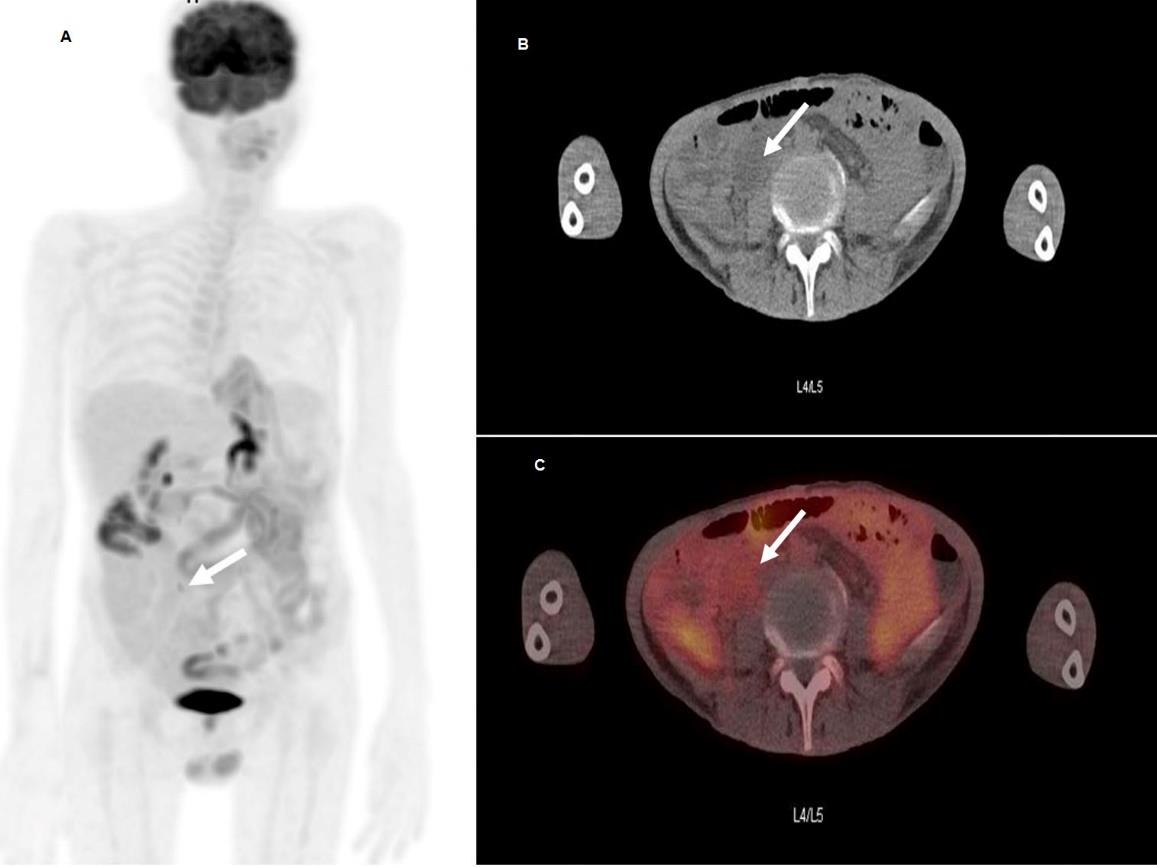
A follow-up ^18^F-FDG PET/CT scan performed 6-month later and showed significant metabolic regression of the previous hypermetabolic segmental lesion (arrows) within the small bowel and with no treatment received in the given time, current SUV_max_: 2.5 compared to previous value of 10. Seen on the on the maximum intensity projection (MIP) image (A), as well as on axial CT (B) and axial fused PET/CT (C) images at the level of L4/L5 vertebrae
